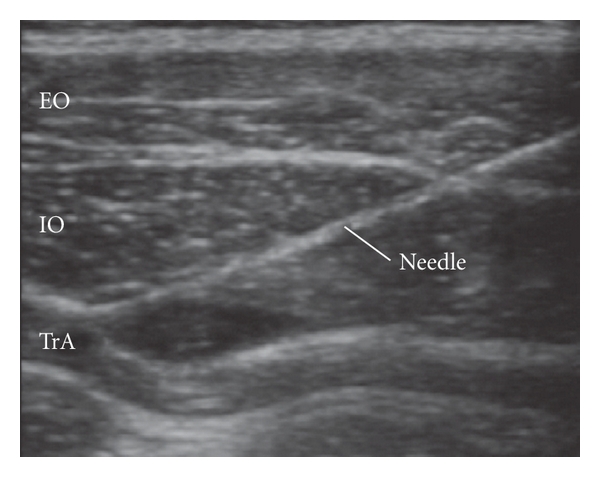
Ultrasound image during initial injection of a small amount of local anesthetic [9]. Reproduced with permission. EO: external oblique, IO: internal oblique, TrA: transversus abdominis.
